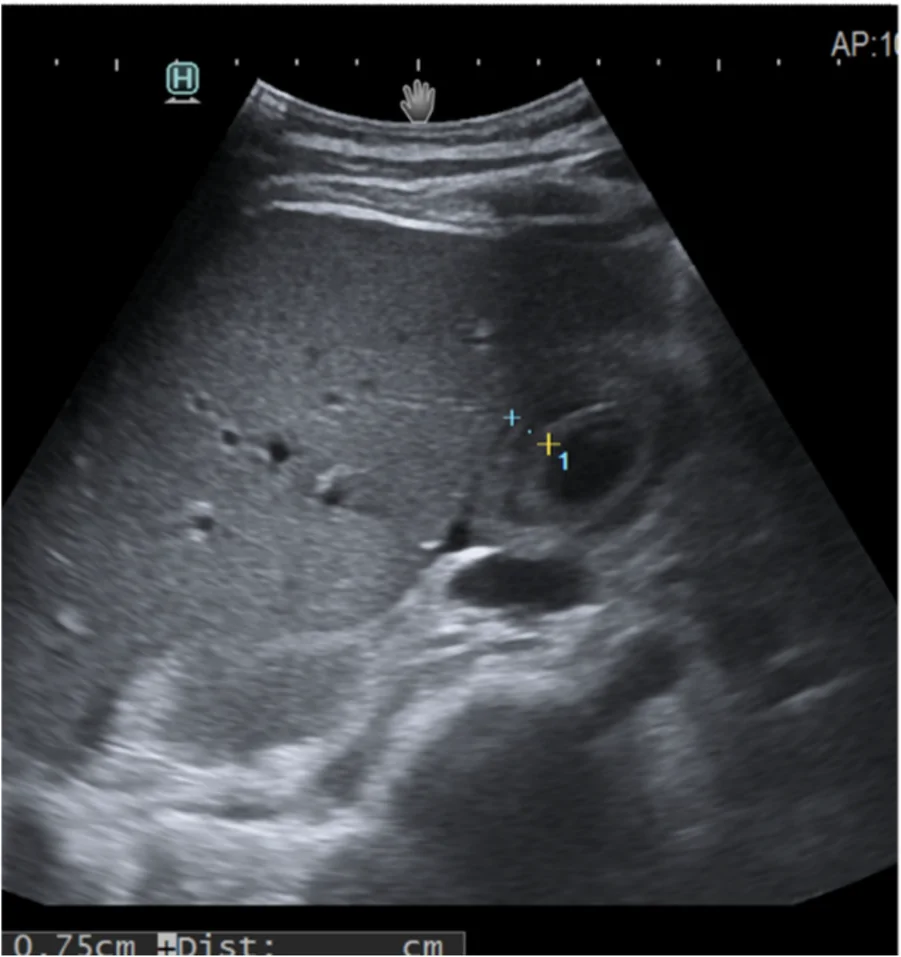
Ultrasound findings suggestive of cholecystitis.
